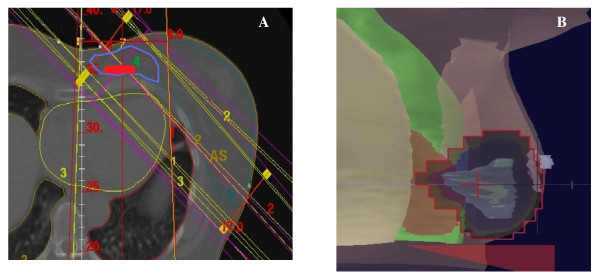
Example of an unfavourable anatomy: inner quadrant tumor (A) on axial CT slice with large lung and heart volume exposure (GTV in red; PTV in blue; heart in pink and ipsilateral lung in blue); (B) Beam Eye View of inner mini-tangent (GTV in blue; PTV in violet; heart in pink; ipsilateral lung in blue; contralateral lung in pale pink).
